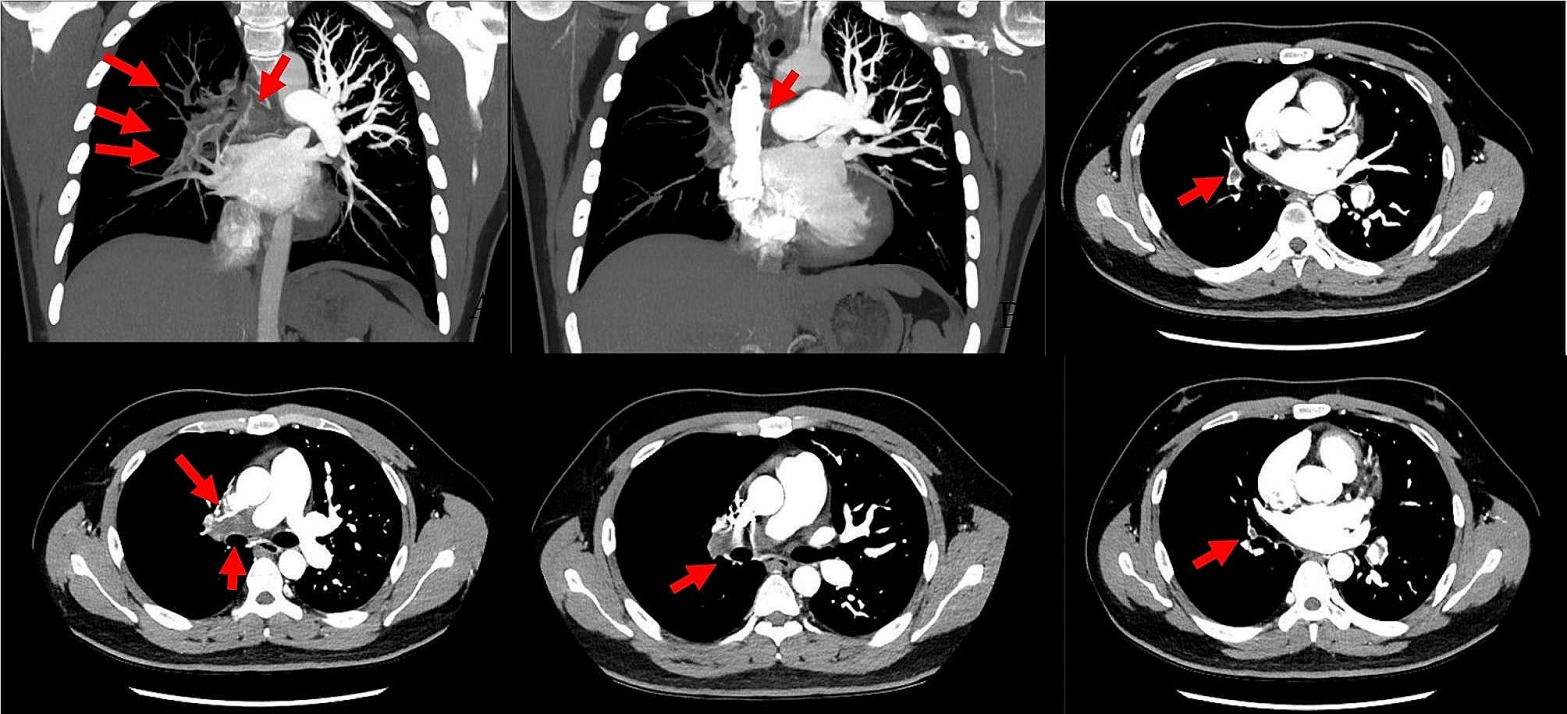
Computed tomographic pulmonary angiography. (A to F) Multiple patchy and lamellar filling defects in the right main pulmonary artery, right upper middle and lower pulmonary artery and its branches
